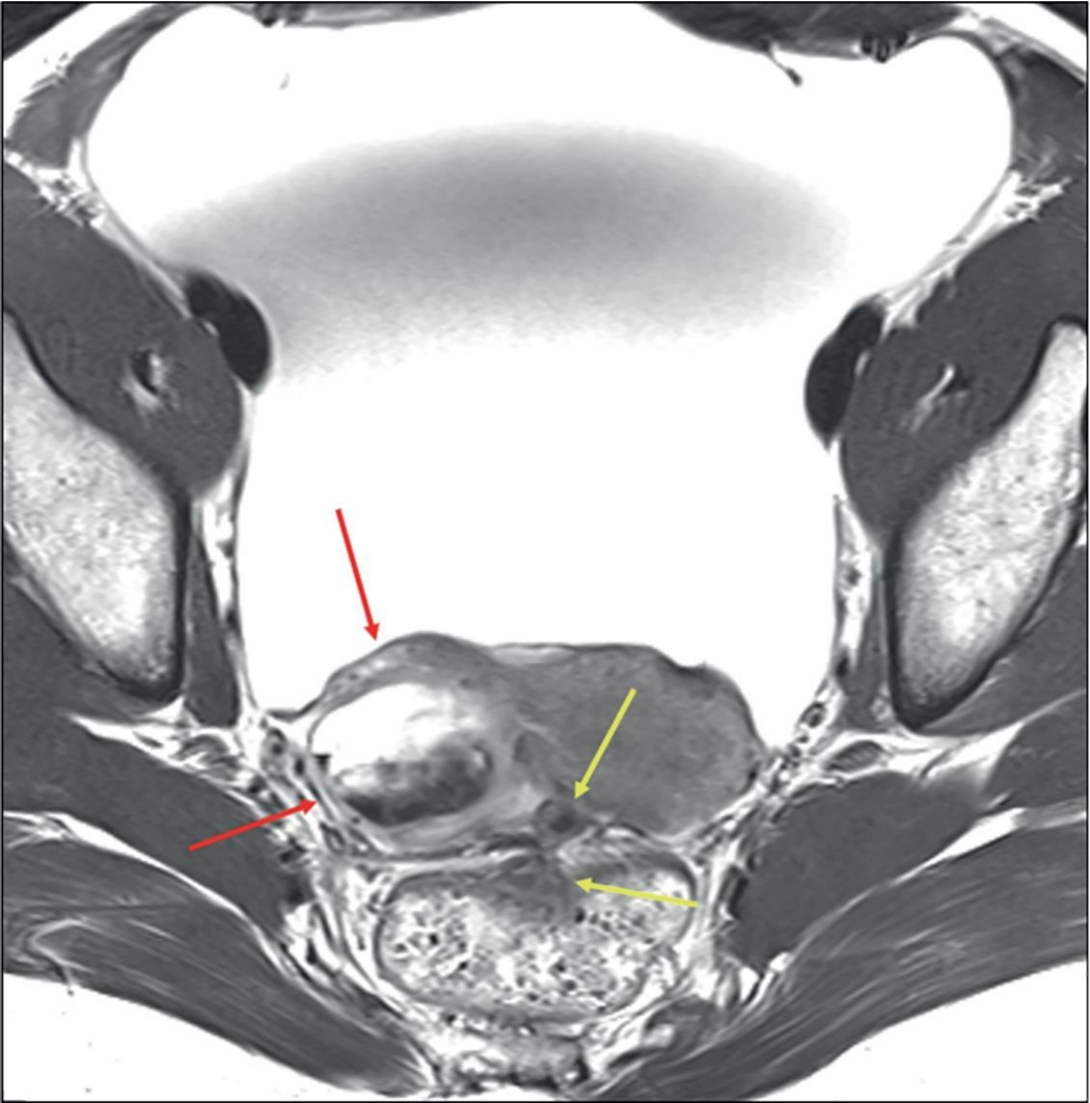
Initial axial T2‐weighted fast spin echo MRI image with findings of endometriosis, including a posterior and medially displaced right ovary with an associated endometrial cyst (red arrows) and deeply infiltrative disease (yellow arrows) obliterating the posterior cul‐de‐sac. Large volume ascites is also noted.
